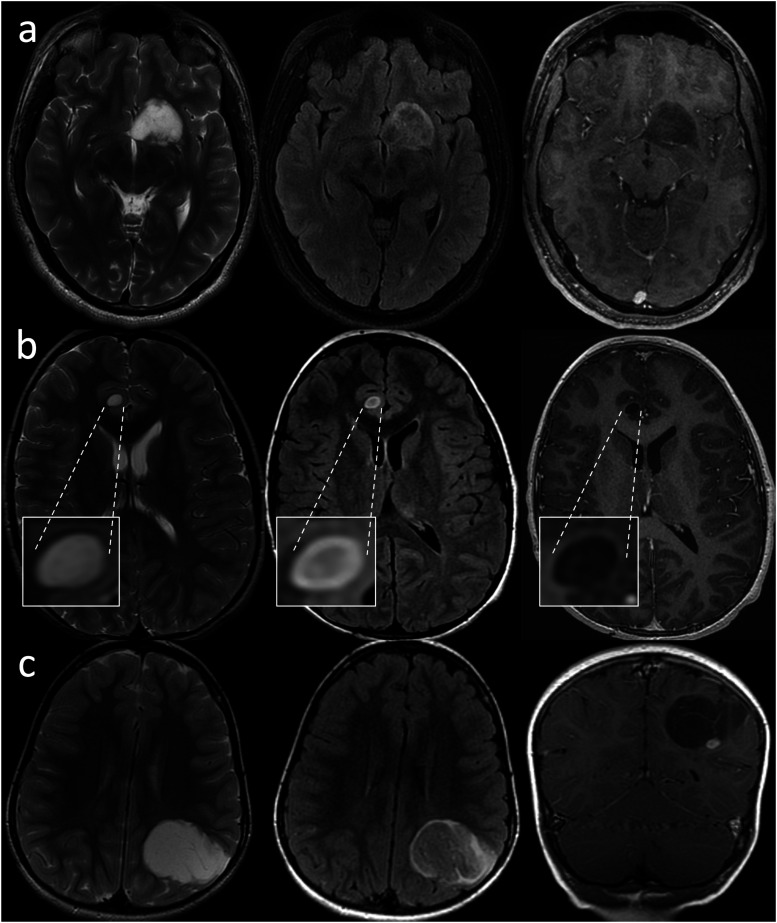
Dysembryoplastic neuroepithelial tumor (DNET) in a 19-year-old male (a), a 6-year-old male (b) and a 5-year-old female (c), diagnosed either via biopsy or resection. (a), (b): The tumors fulfill the strict criteria of the T2FMM sign: complete or near-complete homogeneous hyperintensity on T2WI (left column), relatively hypointense signal on FLAIR except for a thin hyperintense peripheral rim (middle column), and little-to-no enhancement on the postcontrast T1WI (right column). (c): The tumor only partially fulfills the criteria. While the T2WI and FLAIR criteria are met, this lesion has an enhancing nodule as seen on the coronal postcontrast T1WI.
